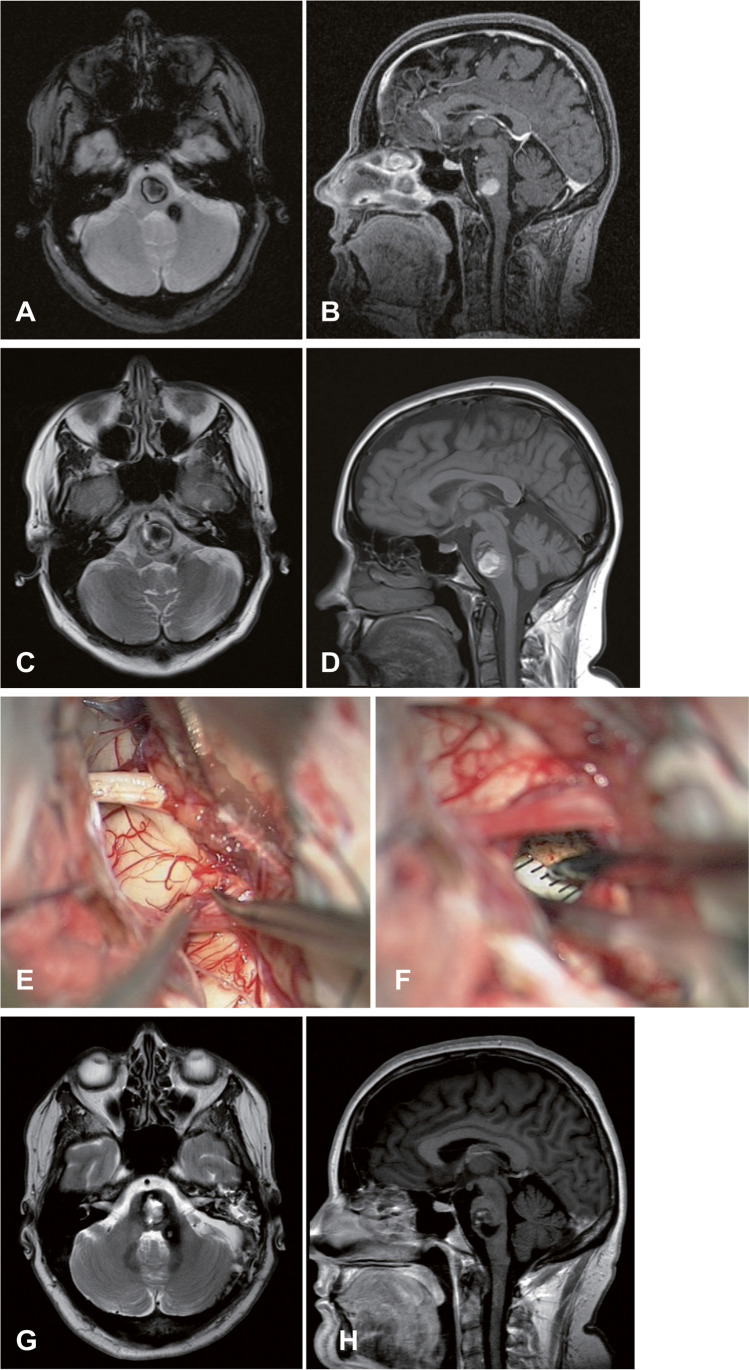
A 44-year-old woman, diagnosed with pontine CM in another hospital due to a hemorrhage event 8 years previously, was referred to our department with dysesthesia of the tongue and fingertips. The MRI scan confirmed multiple intracranial CMs with one large (15 × 21 × 22 mm) in the pons (A and B). The BSCM score was grade VI (size = 2, mRS = 1, hemorrhage event = 2, age = 1, and crossing midline = 0), and conservative treatment was recommended. Two months later, the patient had a recurrent hemorrhage in the pontine CM with right sensorimotor dysfunction, diplopia, and neuroimaging showed enlargement (C and D). The BSCM score was grade X (size = 2, mRS = 4, hemorrhage event = 3, age = 1, and crossing midline = 0). The suboccipital transcondylar approach was used for pontine CM resection (E, F). Postoperative MRI showed partial residual BSCM (G and H). The patient was neurologically intact at the last follow-up 2 years later
